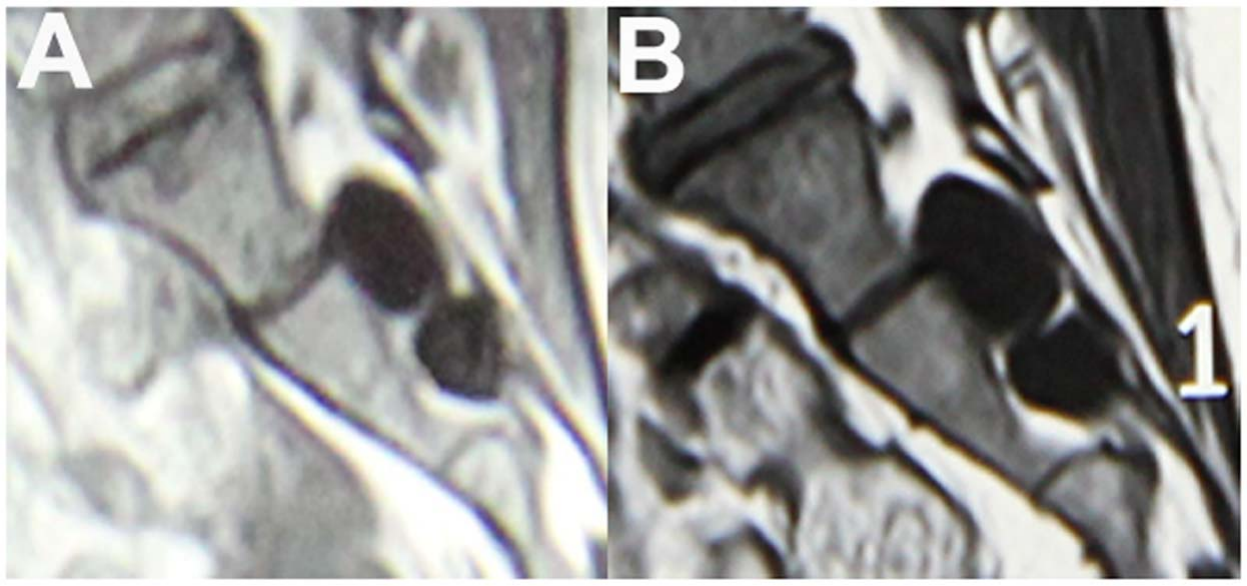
MRI study of progression of the cyst. A: MRI showing two cysts in sacral spinal canal. B: MRI showing that the cysts had grown.
